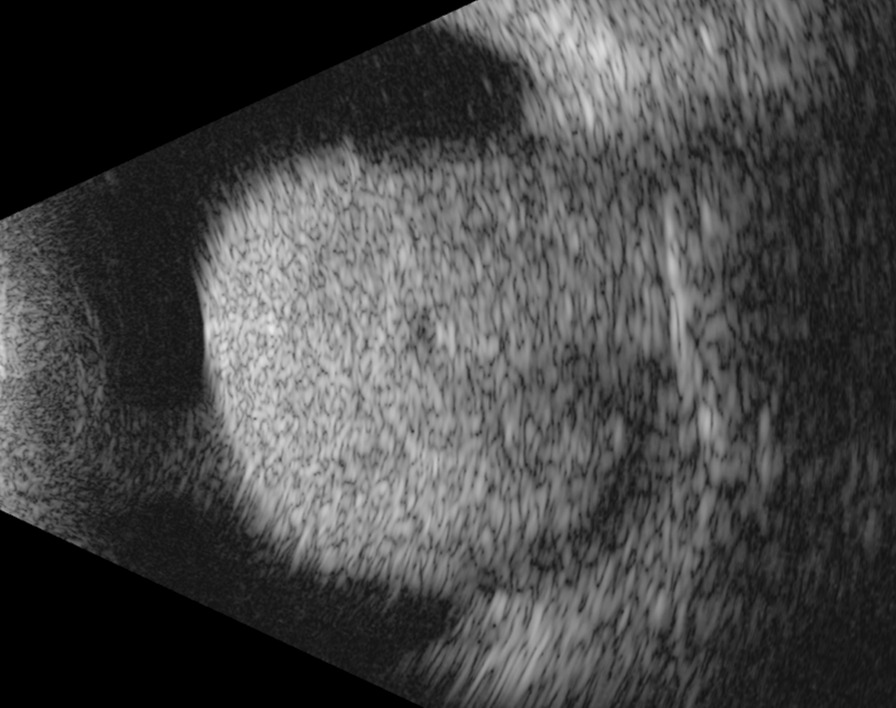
B-scan ultrasound exhibiting a large mushrooming mass of the ciliary body
